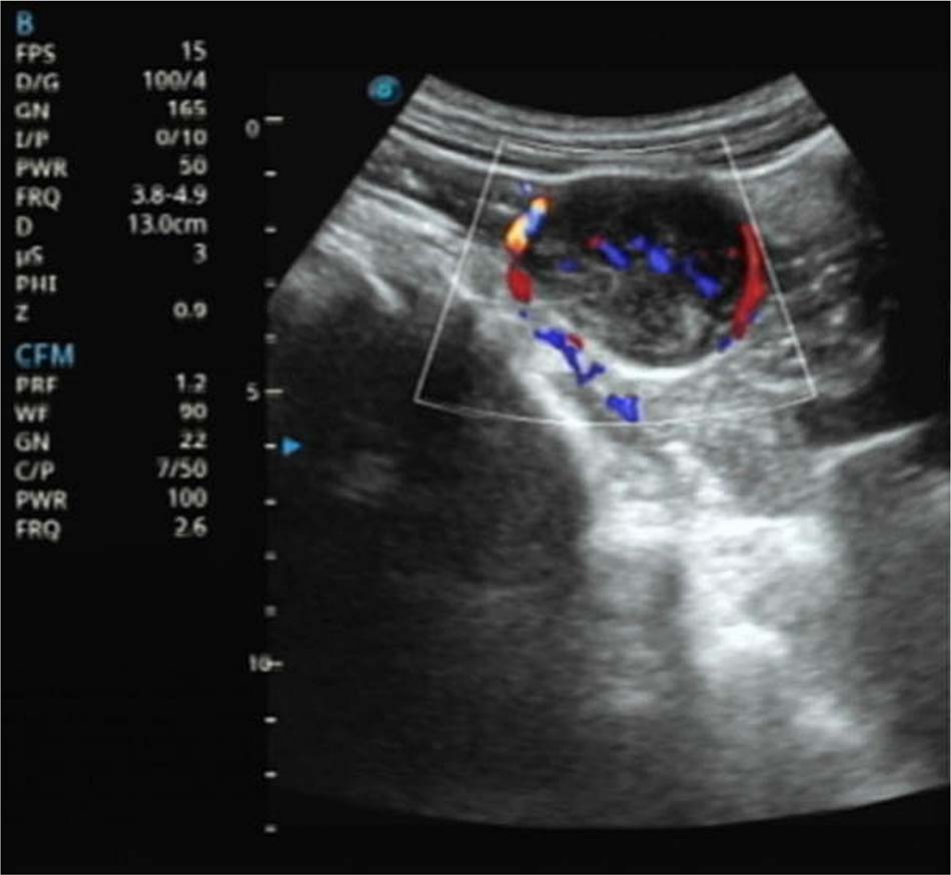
Ultrasonography demonstrates a predominantly hypoechoic mass with peripheral and intralesional blood flow signals.
